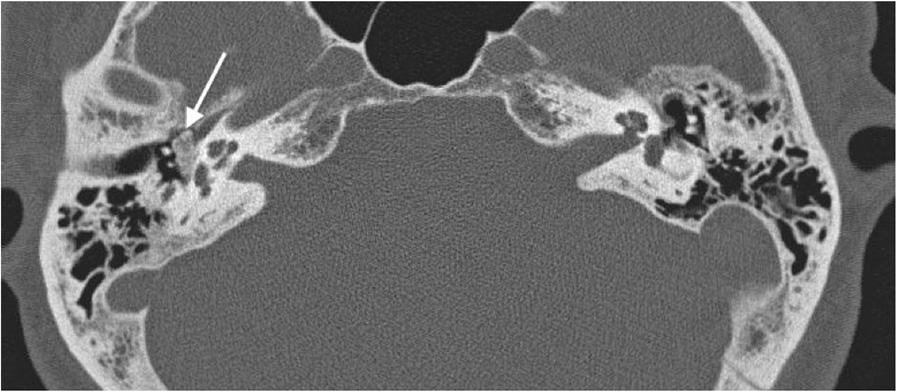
An axial computed tomographic image showing an osseous lesion in the right middle ear space (arrow).
